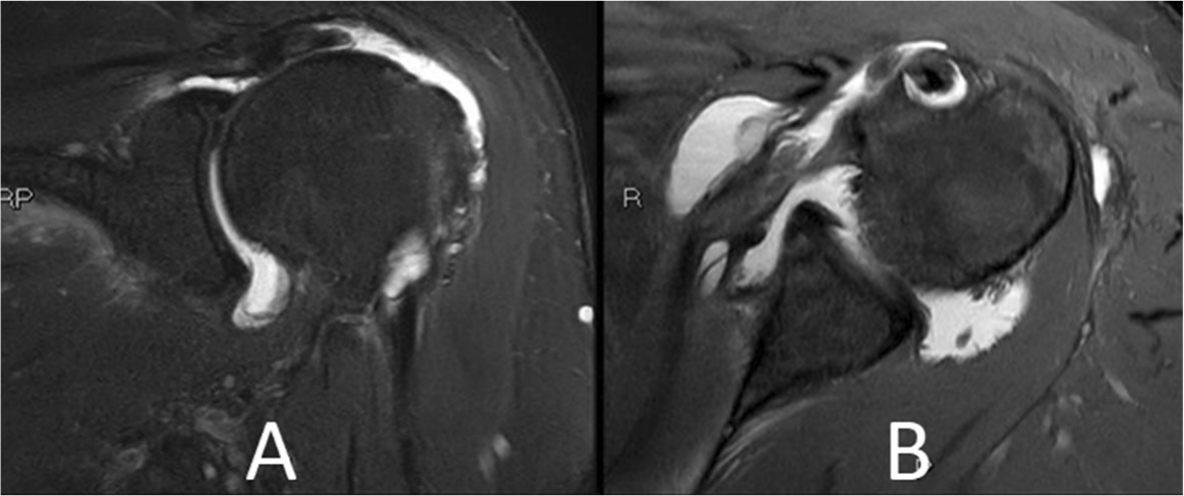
Magnetic Resonance Imaging (MRI) images depicting the retracted full-thickness tear of the supraspinatus tendon (a) and partial-thickness tear of the upper border of the subscapularis tendon (b)
